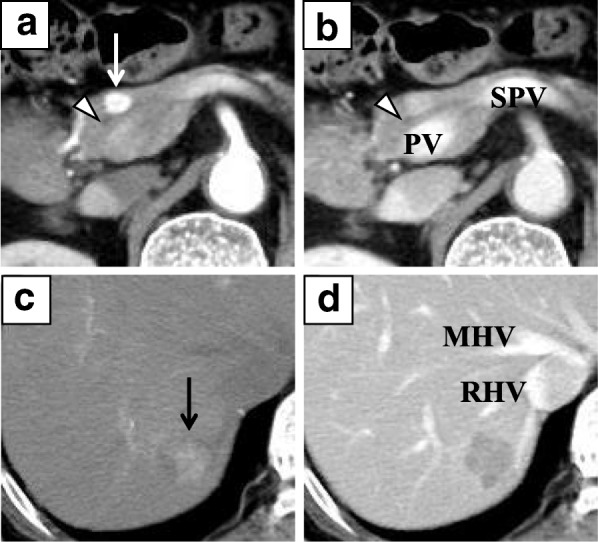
Dynamic CT findings of the pancreatic and liver tumors. a Arterial phase. The pancreatic tumor (white arrow) ventral to the portal vein shows strong enhancement. b Portal phase. The enhancement of the pancreatic tumor is attenuated. Of note, the main pancreatic duct (white arrowhead) is adjacent to the tumor. c Arterial phase. The liver tumor (black arrow) in Couinaud’s segment 7 shows enhancement. d Portal phase. The enhancement of the liver tumor washes out. PV: portal vein, SPV: splenic vein, MHV: middle hepatic vein, RHV: right hepatic vein
